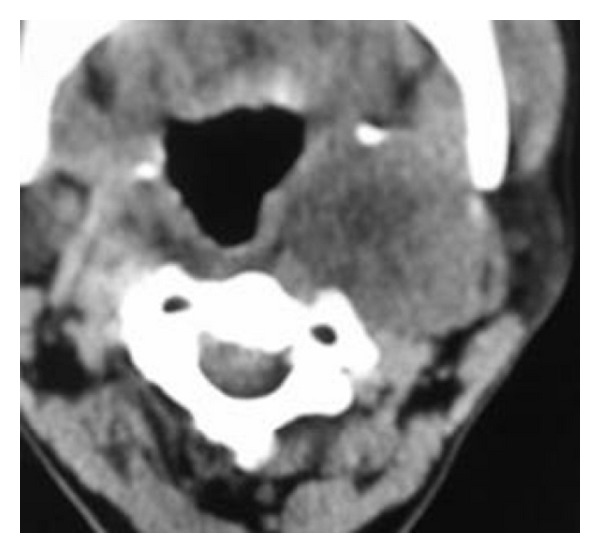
Computed tomography of the neck shows a heterogeneously enhancing hypodense mass on the left carotid space causing splaying of carotid bifurcation.
